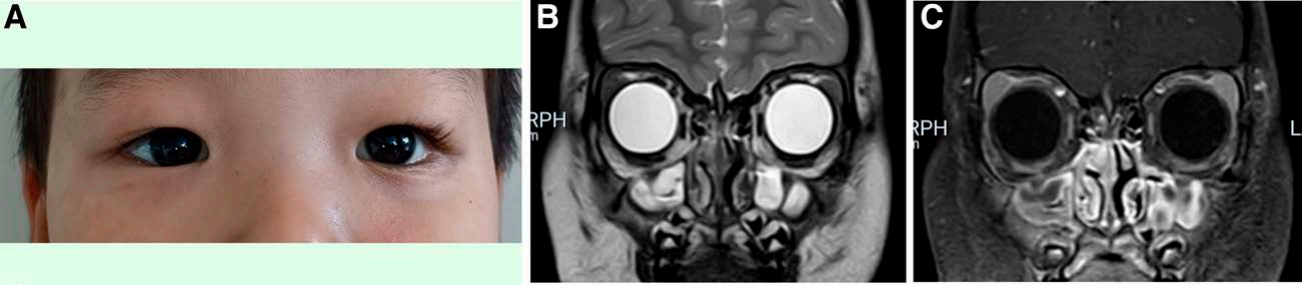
Results of patient review. (A) The appearance of the patient is normal; (B) No tumor recurrence was found on coronal MRI scan on T_2_WI; (C) No tumor recurrence was found on contrast-enhanced T_1_WI. MRI = magnetic resonance imaging, T_1_WI = T_1_ weighted images, T_2_WI = T_2_ weighted images.
